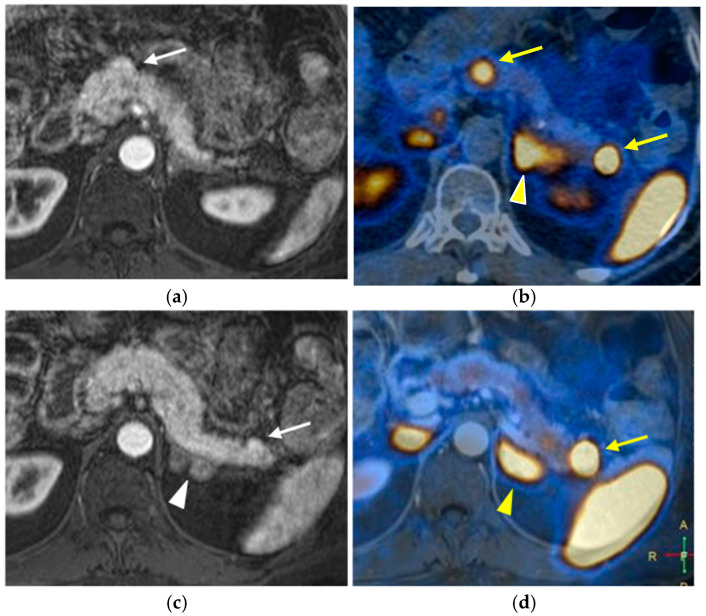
Synchronous non-functioning pancreatic neuroendocrine tumors and adrenal adenomas. Arterial phase contrast-enhanced T1-weighted images show two slightly hyperenhancing nodules (white arrows) in the pancreatic isthmus (a) and in the tail (c). Nodular thickening of the left adrenal gland (white arrowhead) was also found (c). Subsequent 68Ga-DOTATOC-PET-CT (b,d) demonstrated high uptake by the pancreatic (yellow arrows) and adrenal lesions (yellow arrowhead).
